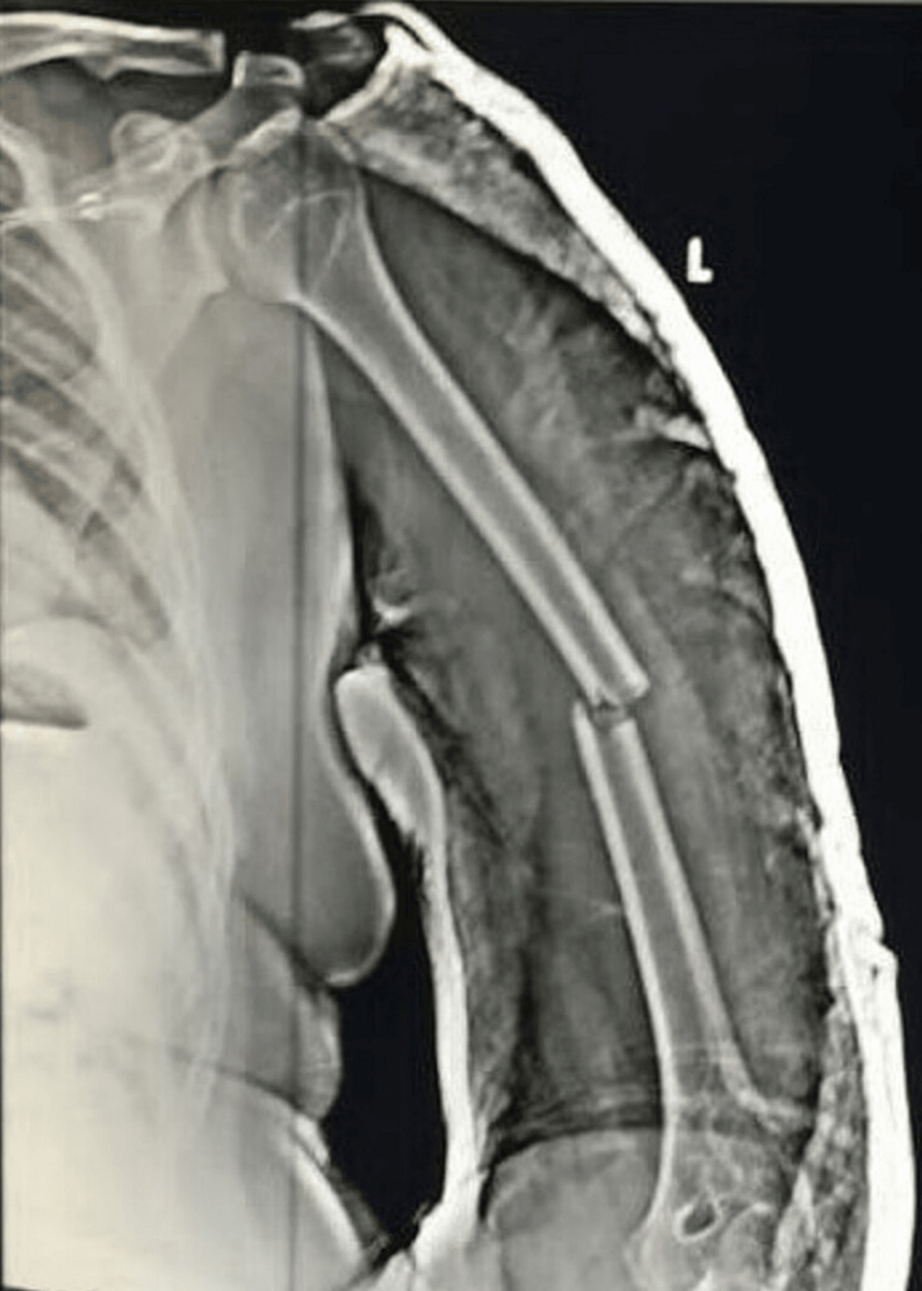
Radiograph taken immediately after reduction using a U slab
